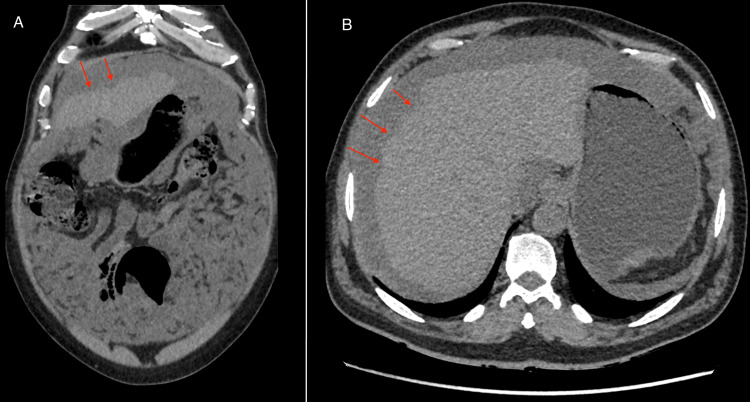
(A) Axial CT scan of the abdomen without contrast, showing hepatic scalloping and mild ascites. (B) Coronal view of the CT scan showing scalloping of the liver. Red arrows in figures A and B indicate the hepatic scalloping, caused by the thick mucinous substances creating indentations on the surface of the liver.
